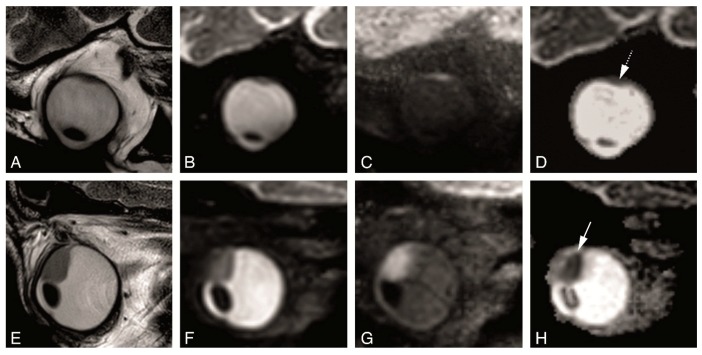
(A–H) TSE DWI in two different patients. (A–D) MS 2 mm sagittal oblique T2 (A), TSE DWI sagittal obliques with b values of 0 s/mm^2^ (B), 800 s/mm^2^ (C) and ADC (D). Restricted diffusion easy to appreciate in a small uveal melanoma (dashed arrow). (E-H) MS 2 mm sagittal oblique T2 (E), TSE DWI sagittal obliques with b values of 0 s/mm^2^ (F), 800 s/mm^2^ (G) and ADC (H). Clear restricted diffusion in the tumor (arrow).
